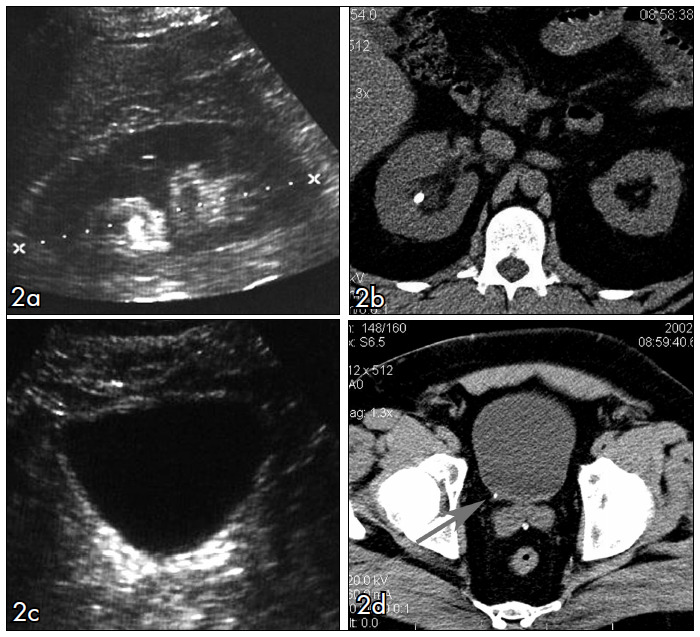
33-year old man with right-sided renal colic. Noncontrast helical computed tomography (CT) and abdominal ultrasound (US) in the same patient. (a) Coronal identification of the right kidney (between calipers) with a renal stone and mild intrarenal collecting system dilatation. The same findings were found on CT scan (b). In the US scan of the bladder and right ureterovesical junction (c), the radiology resident did not find the small stone (3 mm), shown in CT (d).
